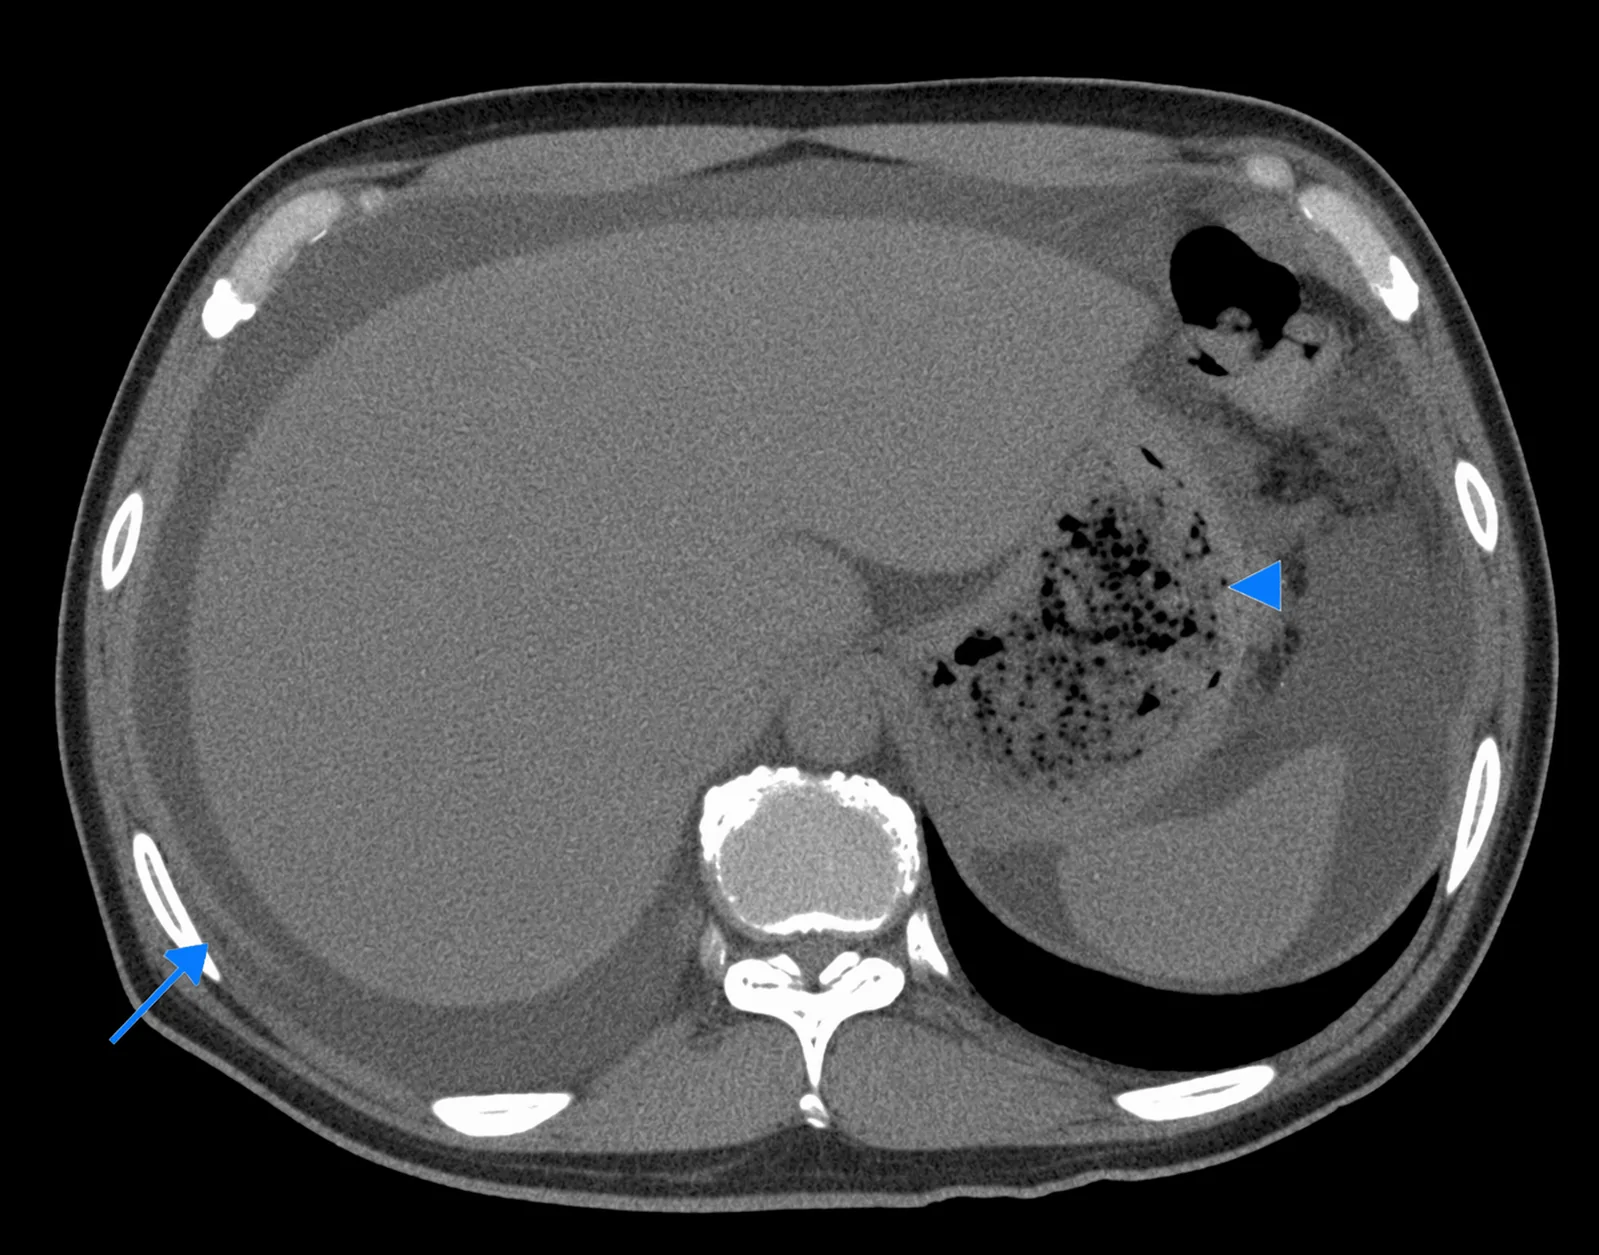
Axial computed tomography (CT) of the abdomen and pelvis without contrast demonstrating moderate abdominopelvic ascites Axial CT of the abdomen and pelvis without contrast demonstrating moderate abdominopelvic ascites (arrow) with diffuse mesenteric congestion (arrowhead), findings consistent with systemic venous congestion.
